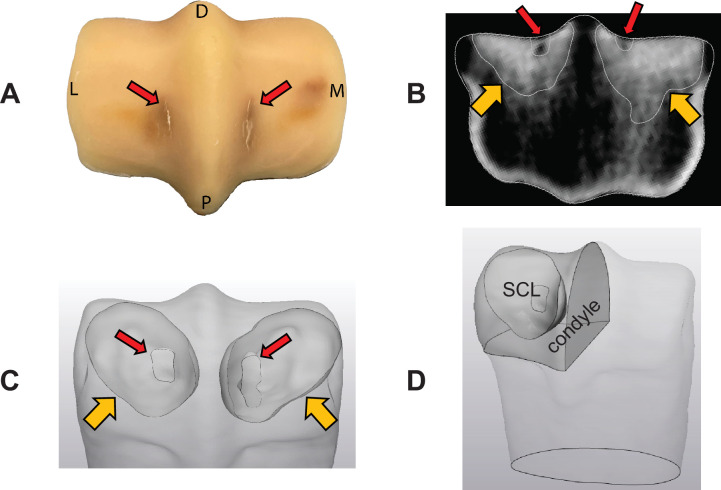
Segmentation of the sclerotic and lytic regions. Adaptive and damage factors were used for tuning Young’s modulus in sclerotic and lytic regions, indicated with orange (wide) and red (thin) arrows, respectively. Fatigue cracks are visible on the palmar aspect of the parasagittal grooves of the condyle PSG-SBI-1 after dissection and removal of soft tissue (A). Sclerotic and lytic regions of PSG-SBI-1 were segmented from the standing CT image (B,C). A representation of the measurement of the volume of a condyle with its sclerotic region is shown (D).
